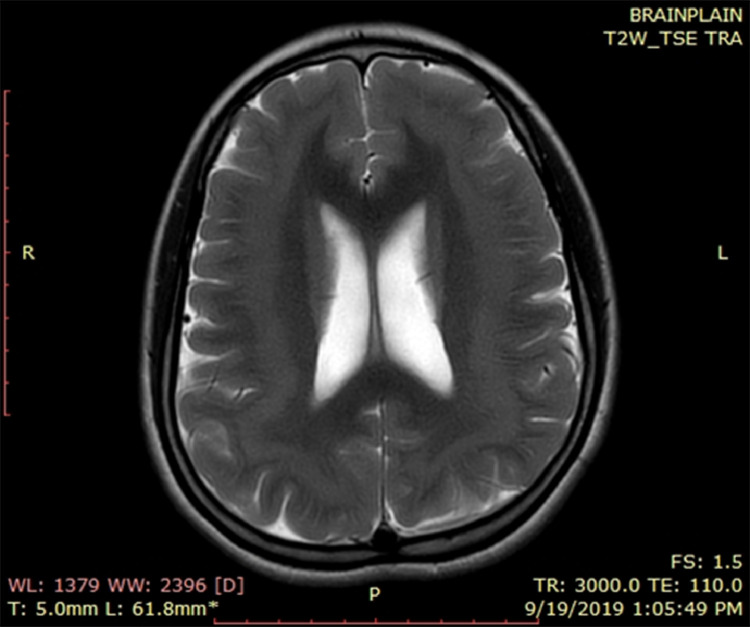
MRI brain plain T2WI showing continuous subcortical band of heterotopic grey matter.
